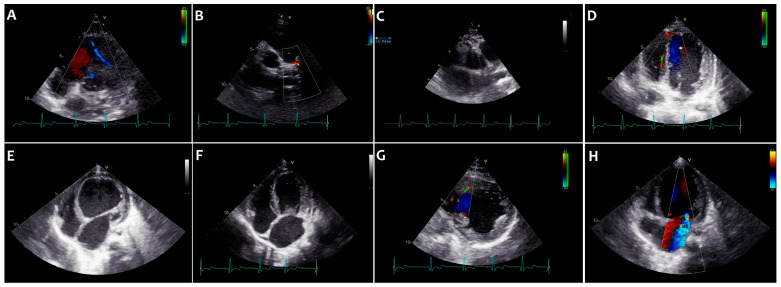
Echocardiographic direct signs: retrograde blood flow in the LCA (#) (A) with associated shunt into the PA (circle) (B). Echocardiographic typical indirect signs: significant RCA dilatation (C) and large coronary collateralization (D,G). Echocardiographic atypical indirect signs: LV dilatation (E), LA dilatation (F), and mitral regurgitation (MR) (H).
